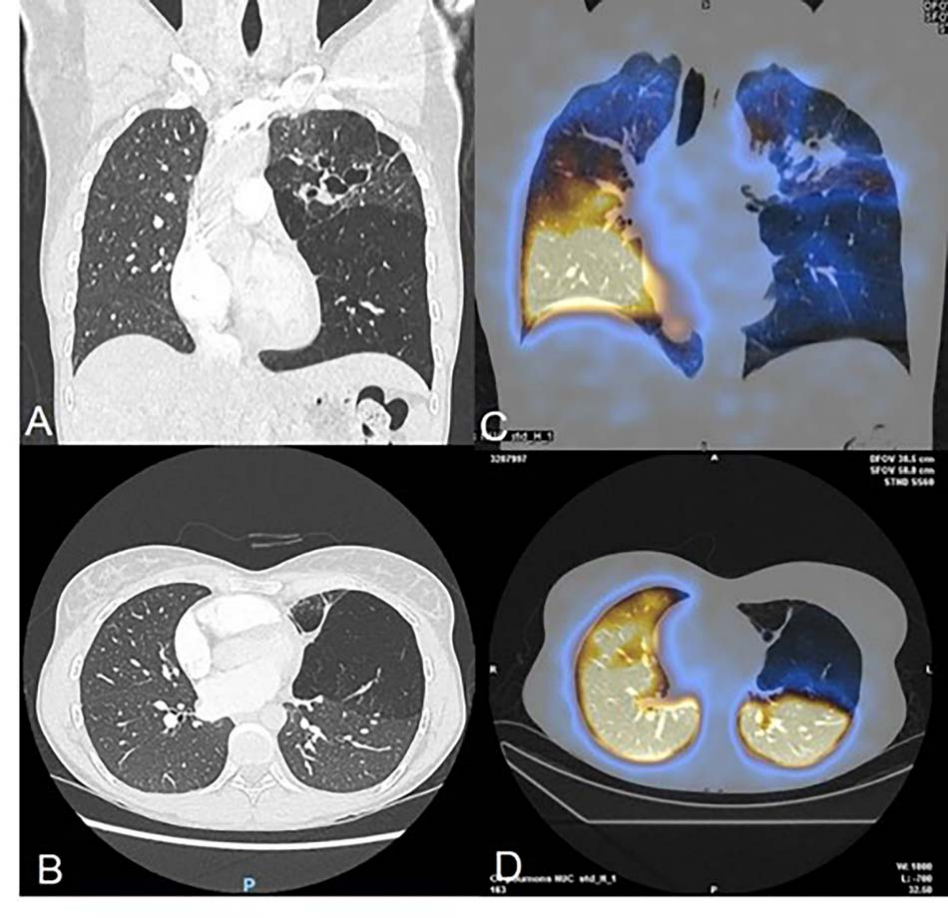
(A–B) Chest CT-scan showing the complex congenital malformation with destruction of the left superior lobe with bronchiectasis and a voluminous bulla of the left lower lobe. (C–D) Perfusion scintigraphy revealing no perfusion of the left upper lobe and bulla of the left lower.
